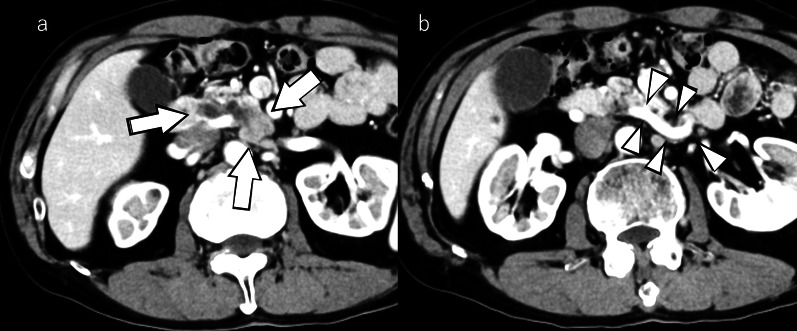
Contrast-enhanced CT of the abdomen. a Cystic lesion with nodules in the uncinated process of the pancreas. b Dilated pancreatic head arcade
